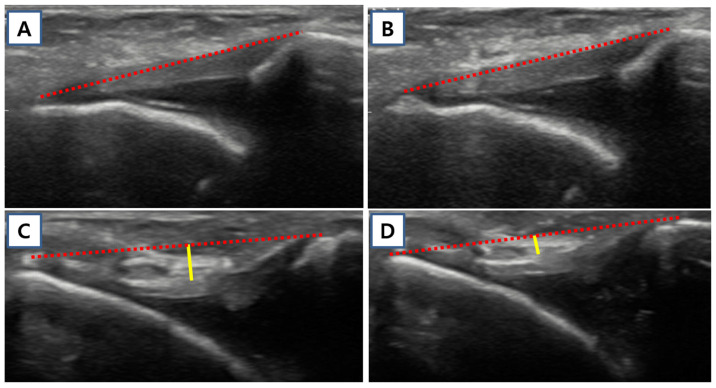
Representative stress ultrasonography images of the anterior talofibular ligament (ATFL). (A,B) A 20-year-old woman from the control group in the resting (A) and stress (B) positions. (C,D) A 26-year-old woman with hEDS in the resting (C) and stress (D) positions. The red dotted line indicates ATFL length and the yellow line indicates ATFL height, representing the degree of ligament laxity.
